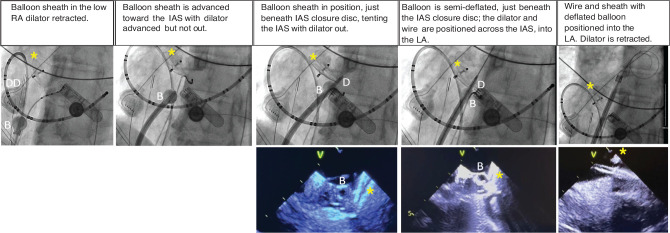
Fluoroscopic and intracardiac echocardiography sequence of balloon transseptal sheath (SafeCross™) used in a very precise interatrial septum (IAS) puncture just beneath an IAS occlusion double disc. The balloon sheath is advanced from the low right atrium up and directed to the IAS in the left anterior oblique view with clear confirmation on intracardiac echocardiography. *Abbreviations:* RA, right atrium; IAS, interatrial septum; LA, left atrium. *Notes:* B, balloon at the distal end of the deflectable transseptal sheath; D, dilator; DD, duadecapolar catheter along the crista terminalis and tricuspid valve isthmus, into the coronary sinus. *IAS double disc closure device.
